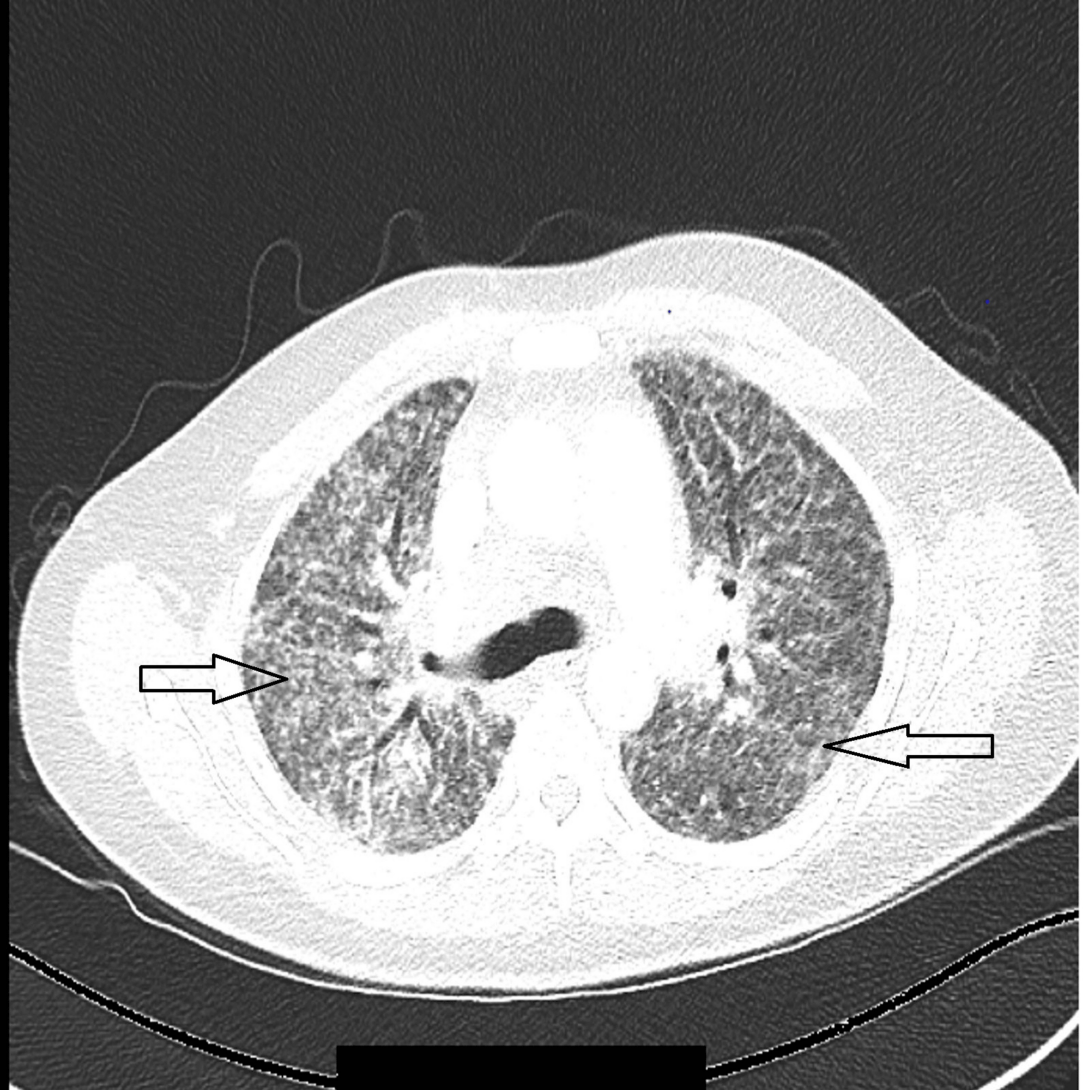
CT scan of the chest, arrows pointed to areas with diffused ground-glass opacification of bilateral lung fields.
